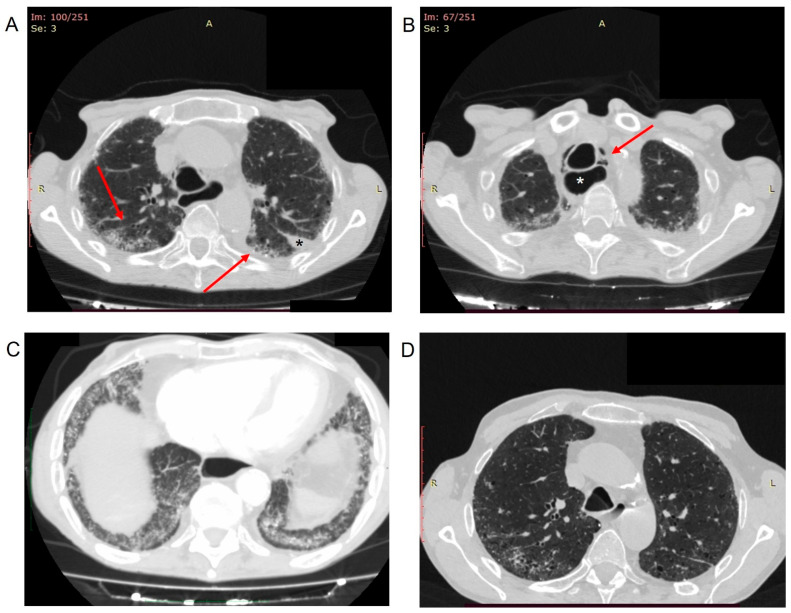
(A–D) Post-COVID-19 computed tomography (CT) pulmonary angiography. (A) Diffuse fibrotic changes with subpleural ground-glass opacity (*arrows*), traction bronchiectasis, and several emphysematous bullae were evident. Mild pleural effusion in the left oblique fissure (*black asterisk*) and mild left-sided paravertebral pleural effusion. (B) Pneumo-mediastinum (*arrow*) with enlargement of the esophagus (*white asterisk*). (C) Mild ground-glass opacification in both lower lobes with sparing of subpleural regions. (D) CT-Scan of the thorax performed two months before the COVID-19, showing peripheral interstitial thickening and traction bronchiectasis due to the systemic sclerosis interstitial lung disease.
